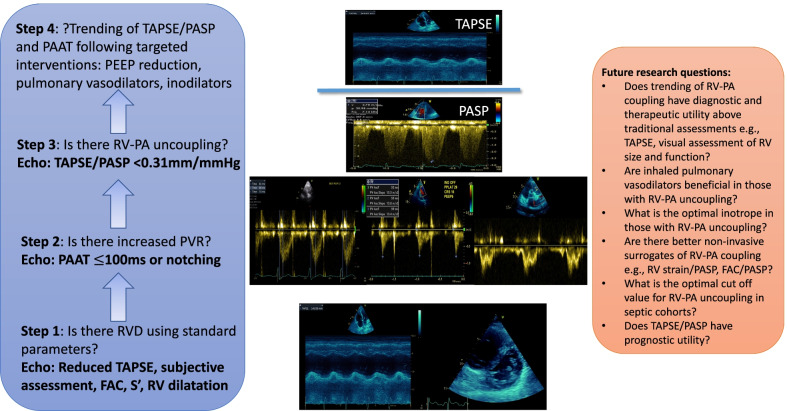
Proposed incremental role of PAAT and TAPSE/PASP in identifying ‘RV dysfunction phenotypes’, i.e. those with concomitant high PVR and/or RV–PA uncoupling. PVR, pulmonary vascular resistance; FAC, fractional area change; S′ = Tissue Doppler RV S′
